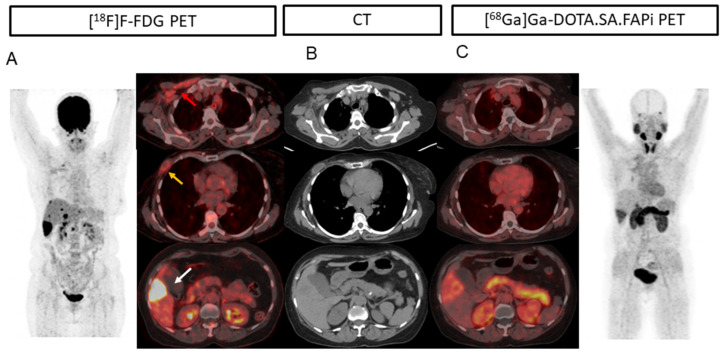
A 56-year-old patient with invasive ductal carcinoma (ER-/PR-/HER2+) underwent neoadjuvant chemotherapy, modified radical mastectomy, capecitabine, and lapatinib chemotherapy. On imaging, [^18^F]F-FDG PET/CT [(A), red arrow] showed uptake in the pectoralis major and minor muscle, which was not observed on [^68^Ga]Ga-DOTA.SA.FAPi PET/CT (C), suggesting inflammation. (A) well-defined cystic lesion with peripheral FDG uptake is noted in the right anterior chest wall at the post-operative site (Right modified radical mastectomy) (yellow arrow) but no uptake was observed on the FAPi scan (B). Compared to [^68^Ga]Ga-DOTA.SA.FAPi PET/CT (B), [^18^F]FDG PET/CT [(A), white arrow] showed intense uptake in the hypodense liver lesion (B) measuring 4.1 × 3 cm in segment VI, subcapsular location. In this patient FAPi could detect all the lesions corresponding to CT, but [^18^F]F-FDG detected false positive findings in the primary site and increased muscle uptake.
